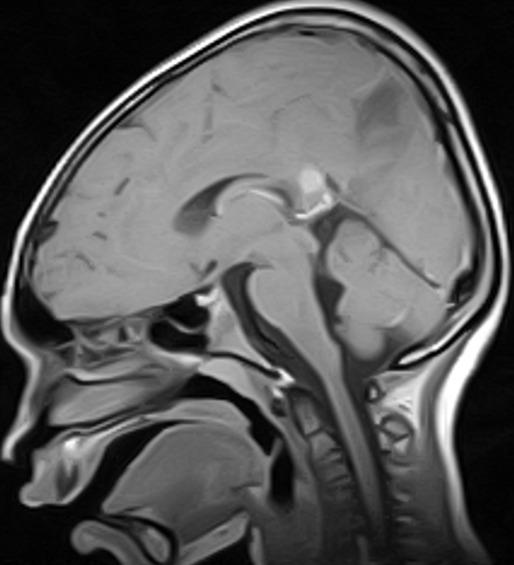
(MRI at presentation) is a T1 w midsagittal sequence showing an irregular hypointense area in the parietal region
